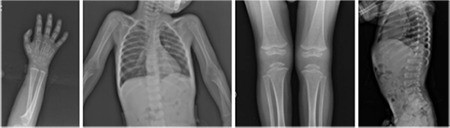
X-ray images show a generalized osteopenia, with epiphyseal dysplasia in the proximal tibia, distal femur, and proximal phalanges, additionally, tubulation deformities in the carpal bones and phalanges
